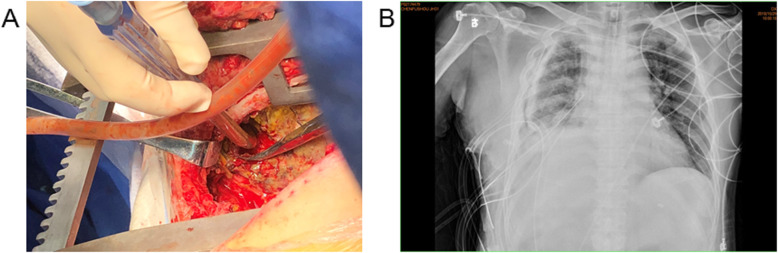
Purulent thoracic clear drainage. a intraoperative observation of the diaphragmatic hole connected to the colon. b postoperative chest X-ray revealed re-expansion of the lung
